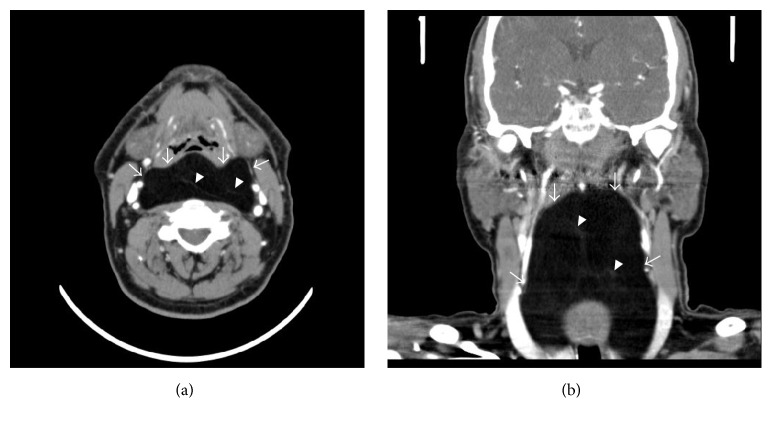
Axial (a) and coronal (b) CT images show a hypodense retropharyngeal mass measuring −80 HU, compatible with lipoma (arrows). Thin regular septations are also seen (arrowheads).
